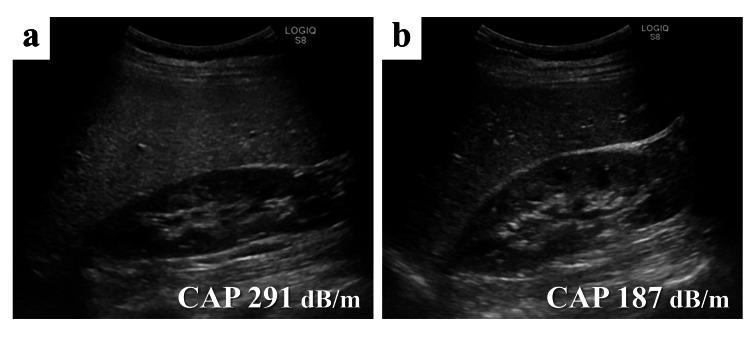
Abdominal images US showing hepato-renal echo contrast on day 28 (a), which disappeared on day 60 (b). CAP, controlled attenuation parameter; US, ultrasonography
